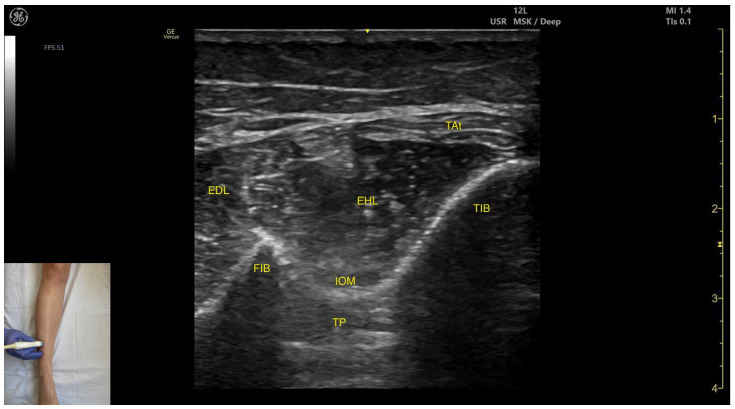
Ultrasound anatomy of the extensor hallucis longus: EDL—extensor digitorum longus; FIB—fibula; EHL—extensor hallucis longus; IOM—interosseous membrane; TP—tibialis posterior; TAt—tibialis anterior tendon; TIB—tibia.
